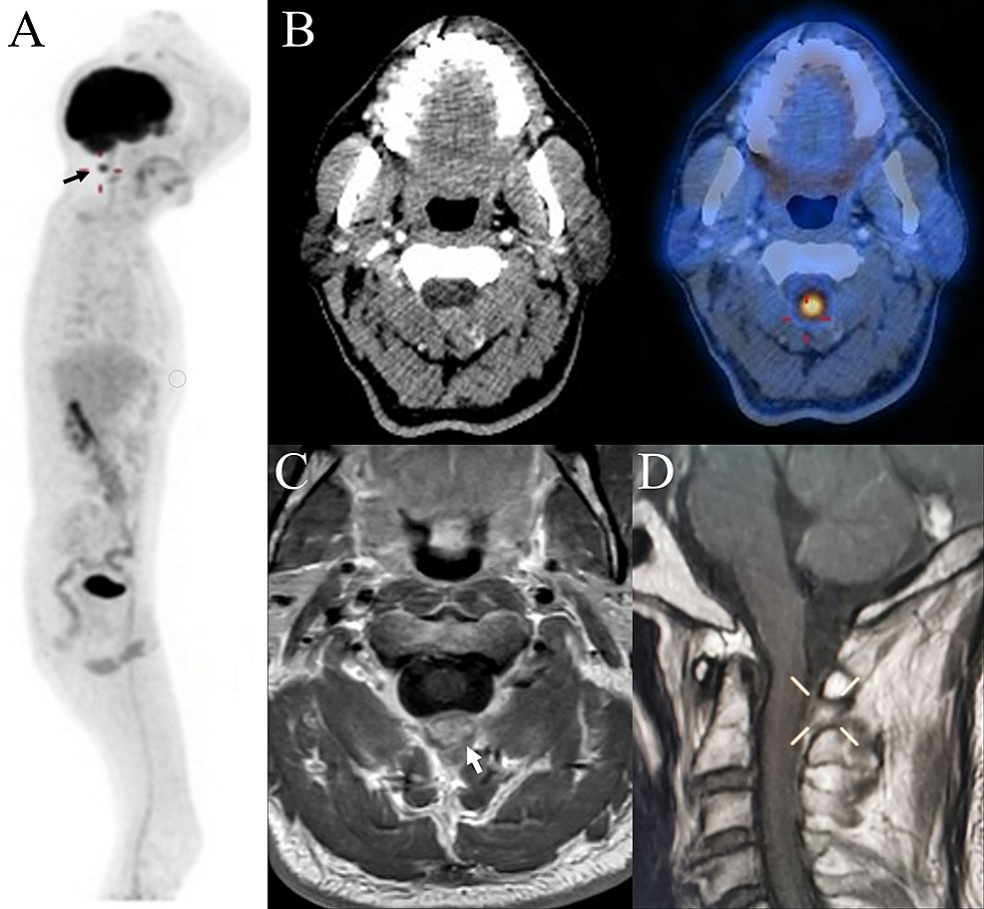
A: Planar lateral view of the 18F-FDG PET/CT showing a hypermetabolic lesion in C2 (black arrow). B: Axial CT scan and 18F-FDG PET/CT scan at C2 level showing the hypermetabolic lesion. C: Axial MRI scan at C2 level showing a hyperintense (1.6 x 0.7 cm) tumor (arrow). D: Sagittal MRI scan showing the hyperintense tumor. 18F-FDG PET/CT, 18F-fluorodeoxyglucose positron emission tomography/computed tomography.
